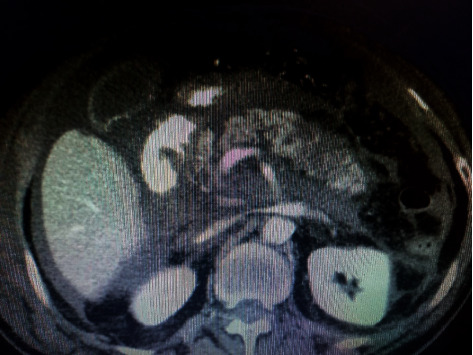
Computed tomography scan of the abdomen showing necrotic areas (hypoenhancing, nonenhancing) in the body of the pancreas.
